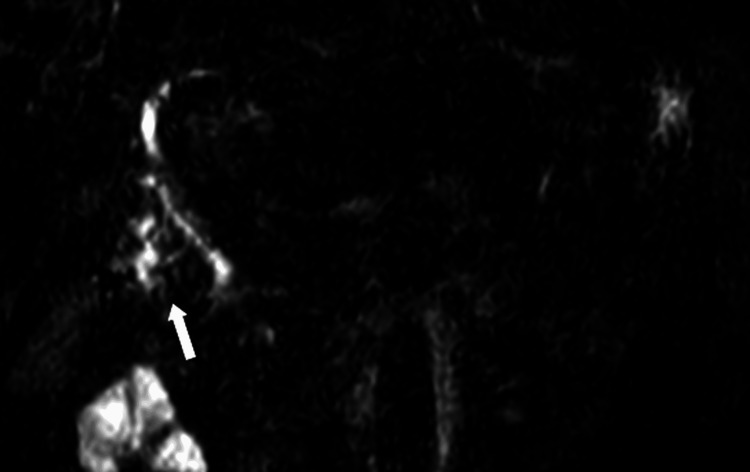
MRCP image of the liver depicting the lesion MRCP image showing marked dilatation of the bile duct in segment VI corresponding to the location of the lesion previously identified on CT (white arrow). MRCP, magnetic resonance cholangiopancreatography.
